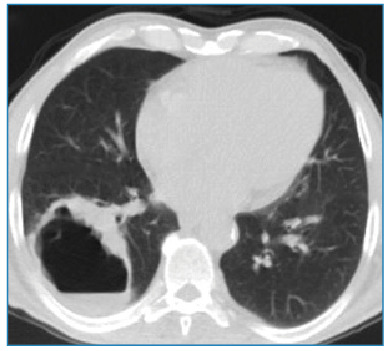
Cavitary lesion in the lower lobe of the right lung on non-contrast thorax computed tomography images.
